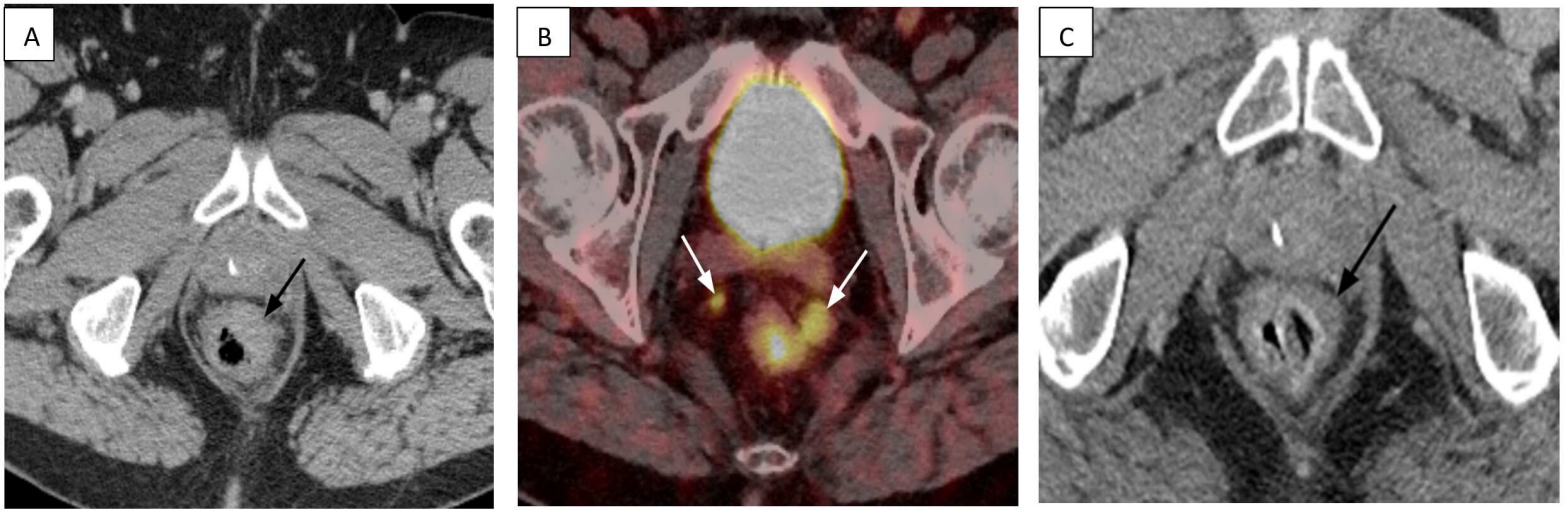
CT and PET/CT imaging of the primary rectal tumor and regional lymph nodes before and after treatment. (A) Baseline axial contrast-enhanced CT scan (July 2024) demonstrates a large, semi-annular soft tissue mass in the low anterior left rectum (black arrow). (B) Staging Gallium-68 dotatate PET/CT (July 2024) reveals dotatate-avid mesorectal lymphadenopathy superior to the primary mass (white arrows). (C) Follow-up axial contrast-enhanced CT scan (March 2025), after systemic therapy, shows a significant treatment response with only minimal residual wall thickening at the tumor site (black arrow) and complete resolution of the lymphadenopathy.
